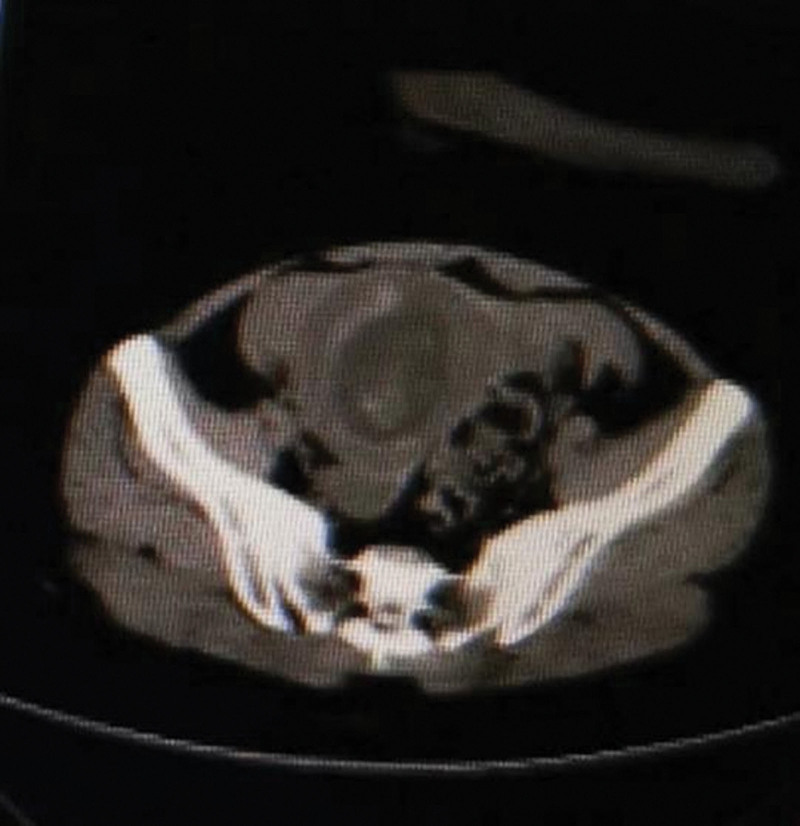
Plain CT scan, fetus in the uterus. A mount of liquid was stored in abdominal cavity.
